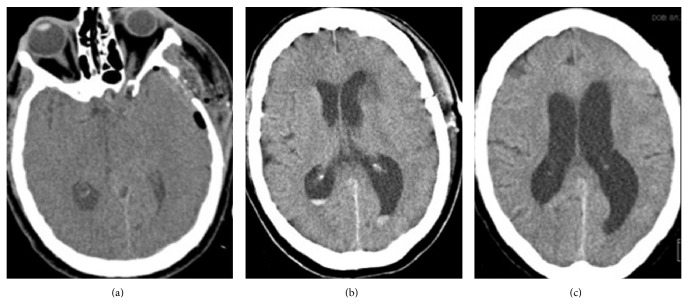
(a) Noncontrast axial computed tomography scan of the head done on postoperative day 5 showing some postoperative changes after a left frontotemporal craniotomy for resection of the patient's recurrent planum sphenoidale meningioma. The scan did not show any intraventricular hemorrhage; however, it showed unilateral enlargement of the left lateral ventricle. (b) Noncontrast axial computed tomography scan of the head done one day after the epidural blood patch showing a new-onset hemorrhage layering the occipital horns of both lateral ventricles. (c) Noncontrast axial head computed tomography scan done two weeks later showing complete resolution of the intraventricular hemorrhage.
